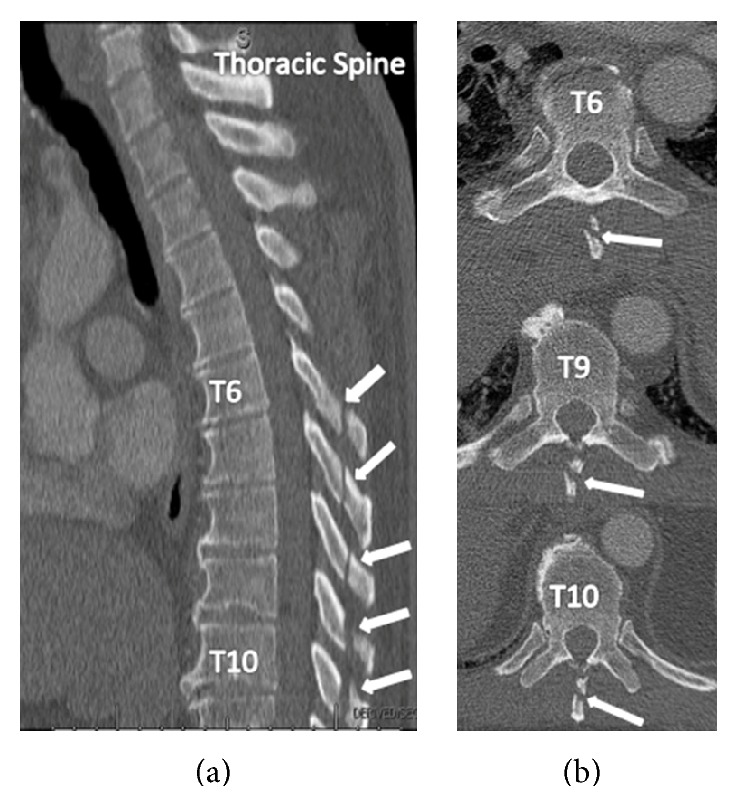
(a) Sagittal CT demonstrating thoracic spinous process fractures (arrows) spanning from T6 to T10. (b) Axial CT showing select (T6, T9, and T10) spinous process fractures (arrows). No evidence of vertebral body or intralaminar extension is noted.
